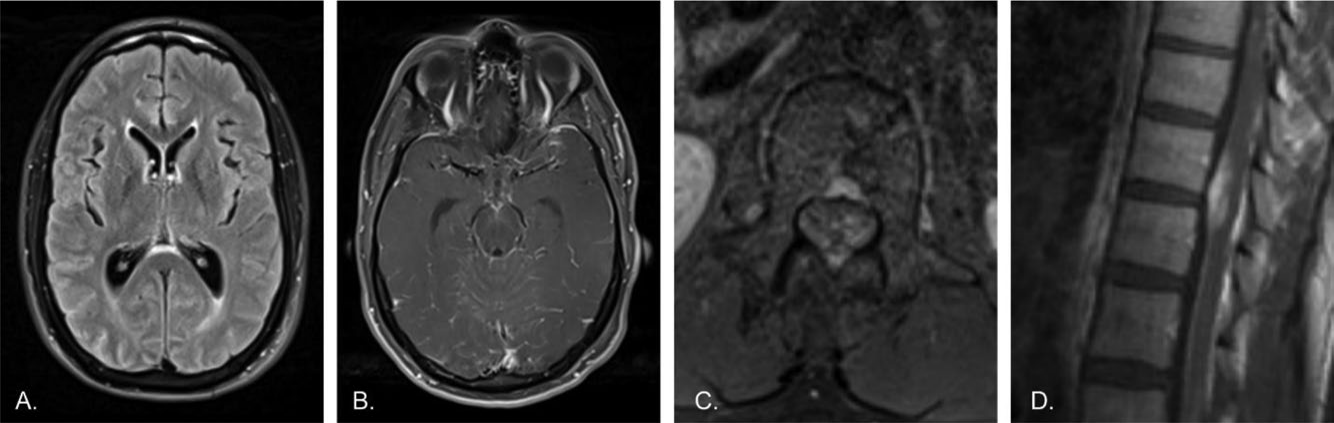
A previously healthy 33-year-old male presents with new-onset encephalopathy, headaches, and seizures. A) Axial T2-FLAIR image of the brain shows slight periventricular and sulcal hyperintensity. B) Axial post-contrast T1 image of the brain shows diffuse subtle leptomeningeal enhancement, including along the midbrain surface and cerebellar folia. There was no associated diffusion restriction (not shown). C) Axial post-contrast T1 image of the lumbar spine shows enhancement of the cauda equina and about the conus. D) Sagittal post-contrast T1 image of the lower thoracic spine shows diffuse enhancement of the spinal cord surface with more mass-like enhancement involving the ventral cord.
